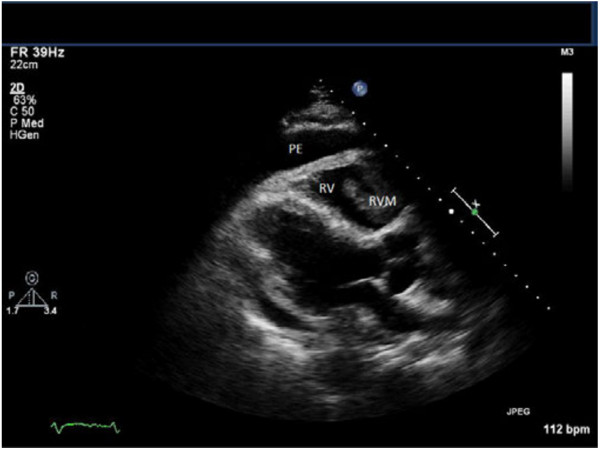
Transthoracic Echocardiogram in a Parasternal Long Axis View showing a Large Pericardial effusion and RV Mass Large Pericardial effusion and RV Mass.
